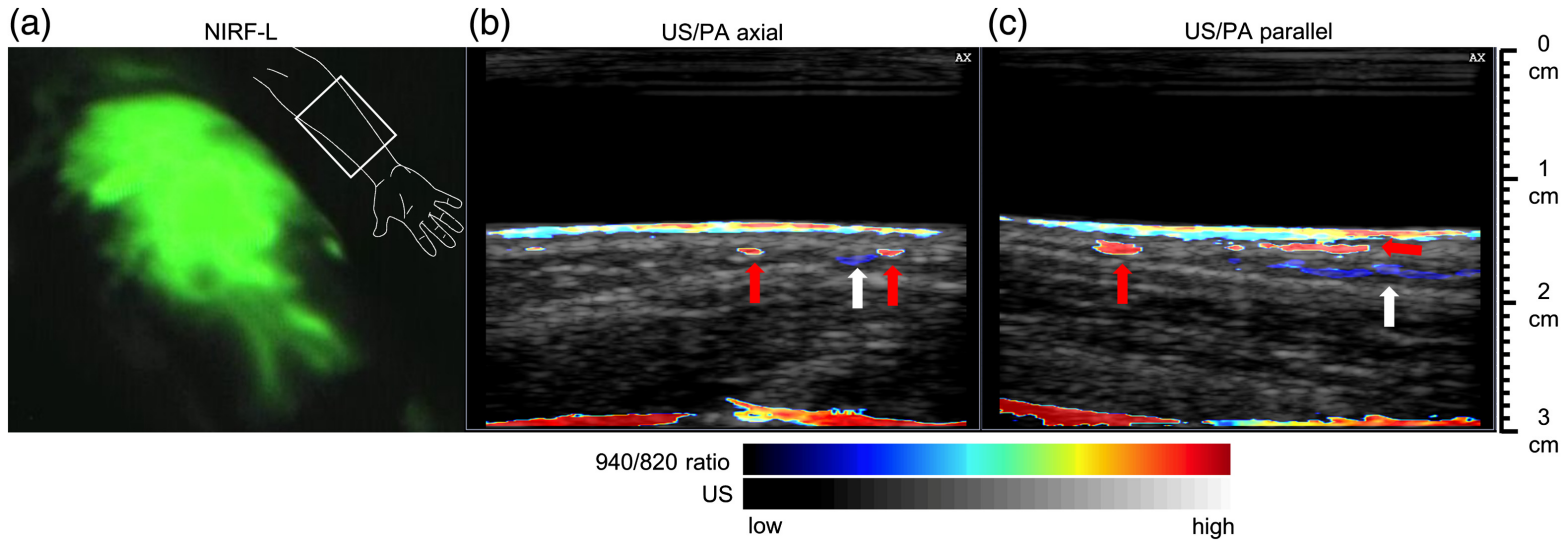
PA images of lymphatic vessels and veins overlaid on ultrasound (US) images. (a) NIRF-L image of a dorsal forearm, showing extensive dermal backflow that limits the visibility of linear lymphatic vessels. (b) and (c) Corresponding PA images overlaid on ultrasound in an axial and parallel position of the probe, red and white arrows indicate the veins and lymphatic vessels. Adapted from Ref. 168, available under a CC-BY 4.0 license.
